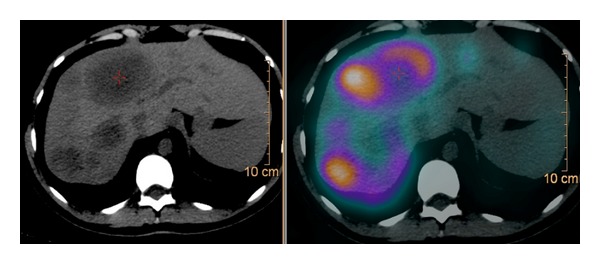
Octreoscan SPECT/CT after 1st infusion of radiolabeled somatostatin analog lutetium (^177^LU) administration.
